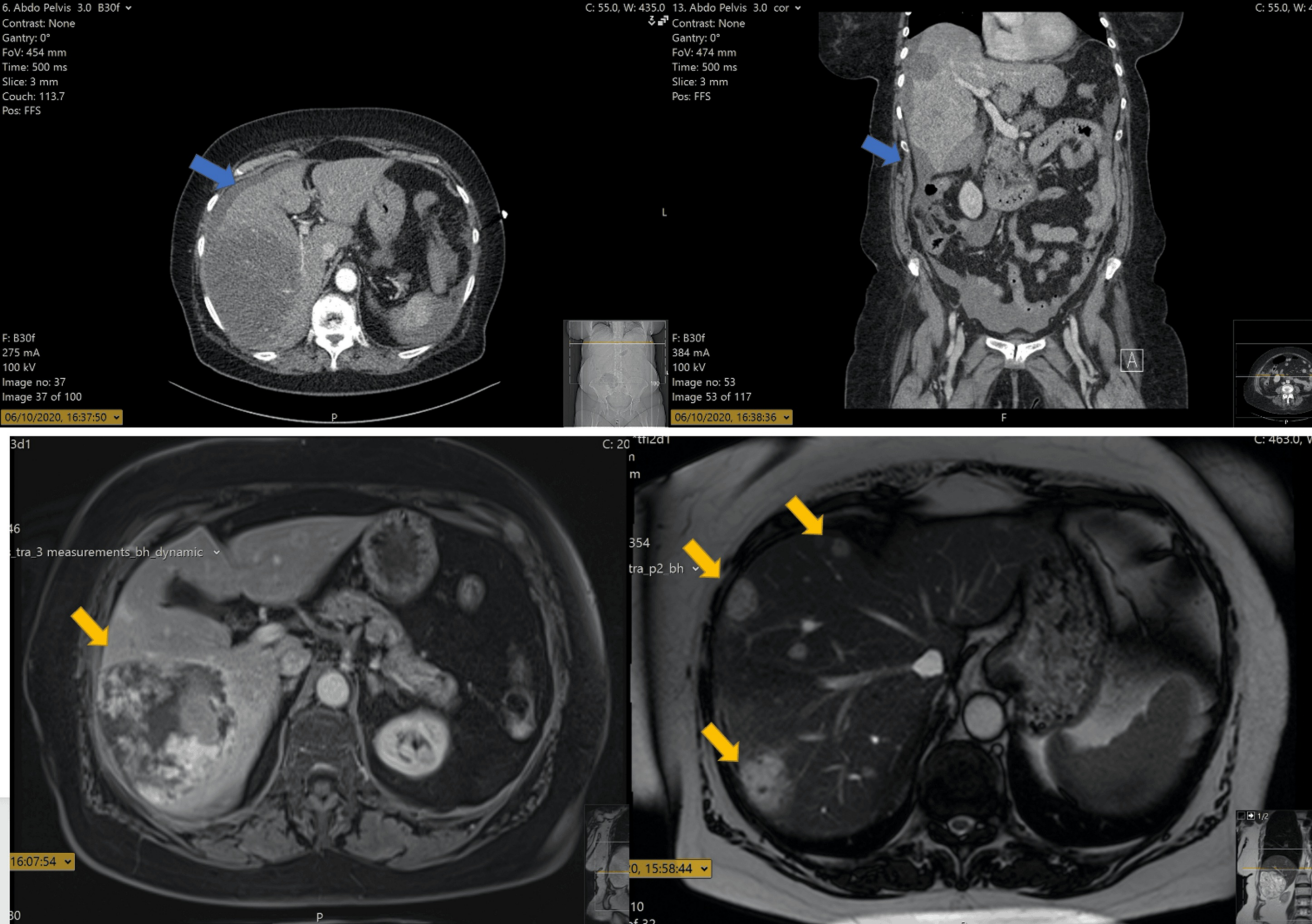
HA mimicking cavernous haemangioma On top, CECT demonstrates spontaneous HA bleeding with perihepatic free fluid evident (blue arrows). On the bottom, MRI imaging shows HA progression in size but also new lesions demonstrating disease spread. MRI: magnetic resonance imaging; HA: hepatic angiosarcoma; CECT: contrast-enhanced computed tomography
